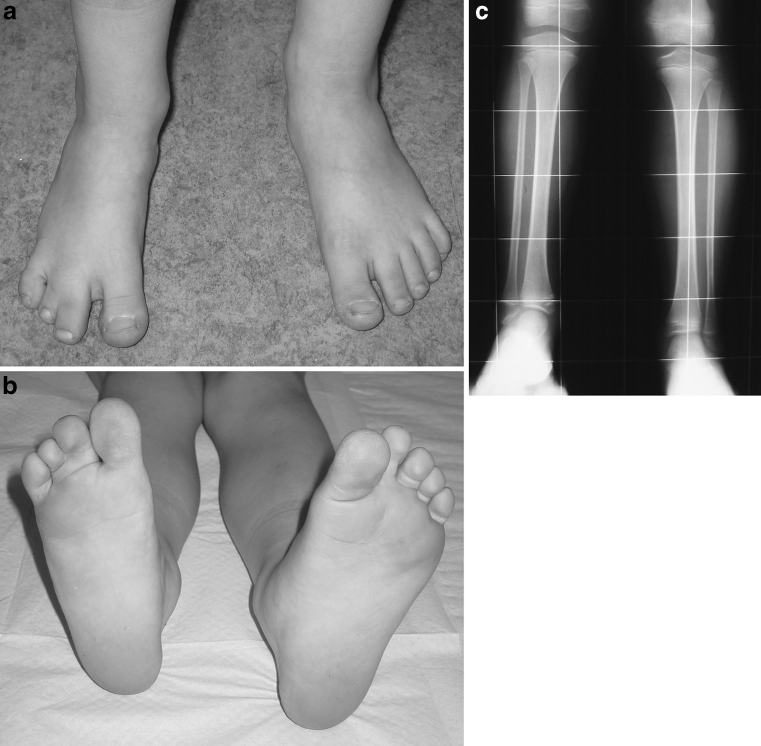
Right foot hypoplasia with a normal shape of both the lateral and the medial foot ray (a, b). X-ray with slight shortening, a normal fibula and a ball-and-socket ankle joint (c)
